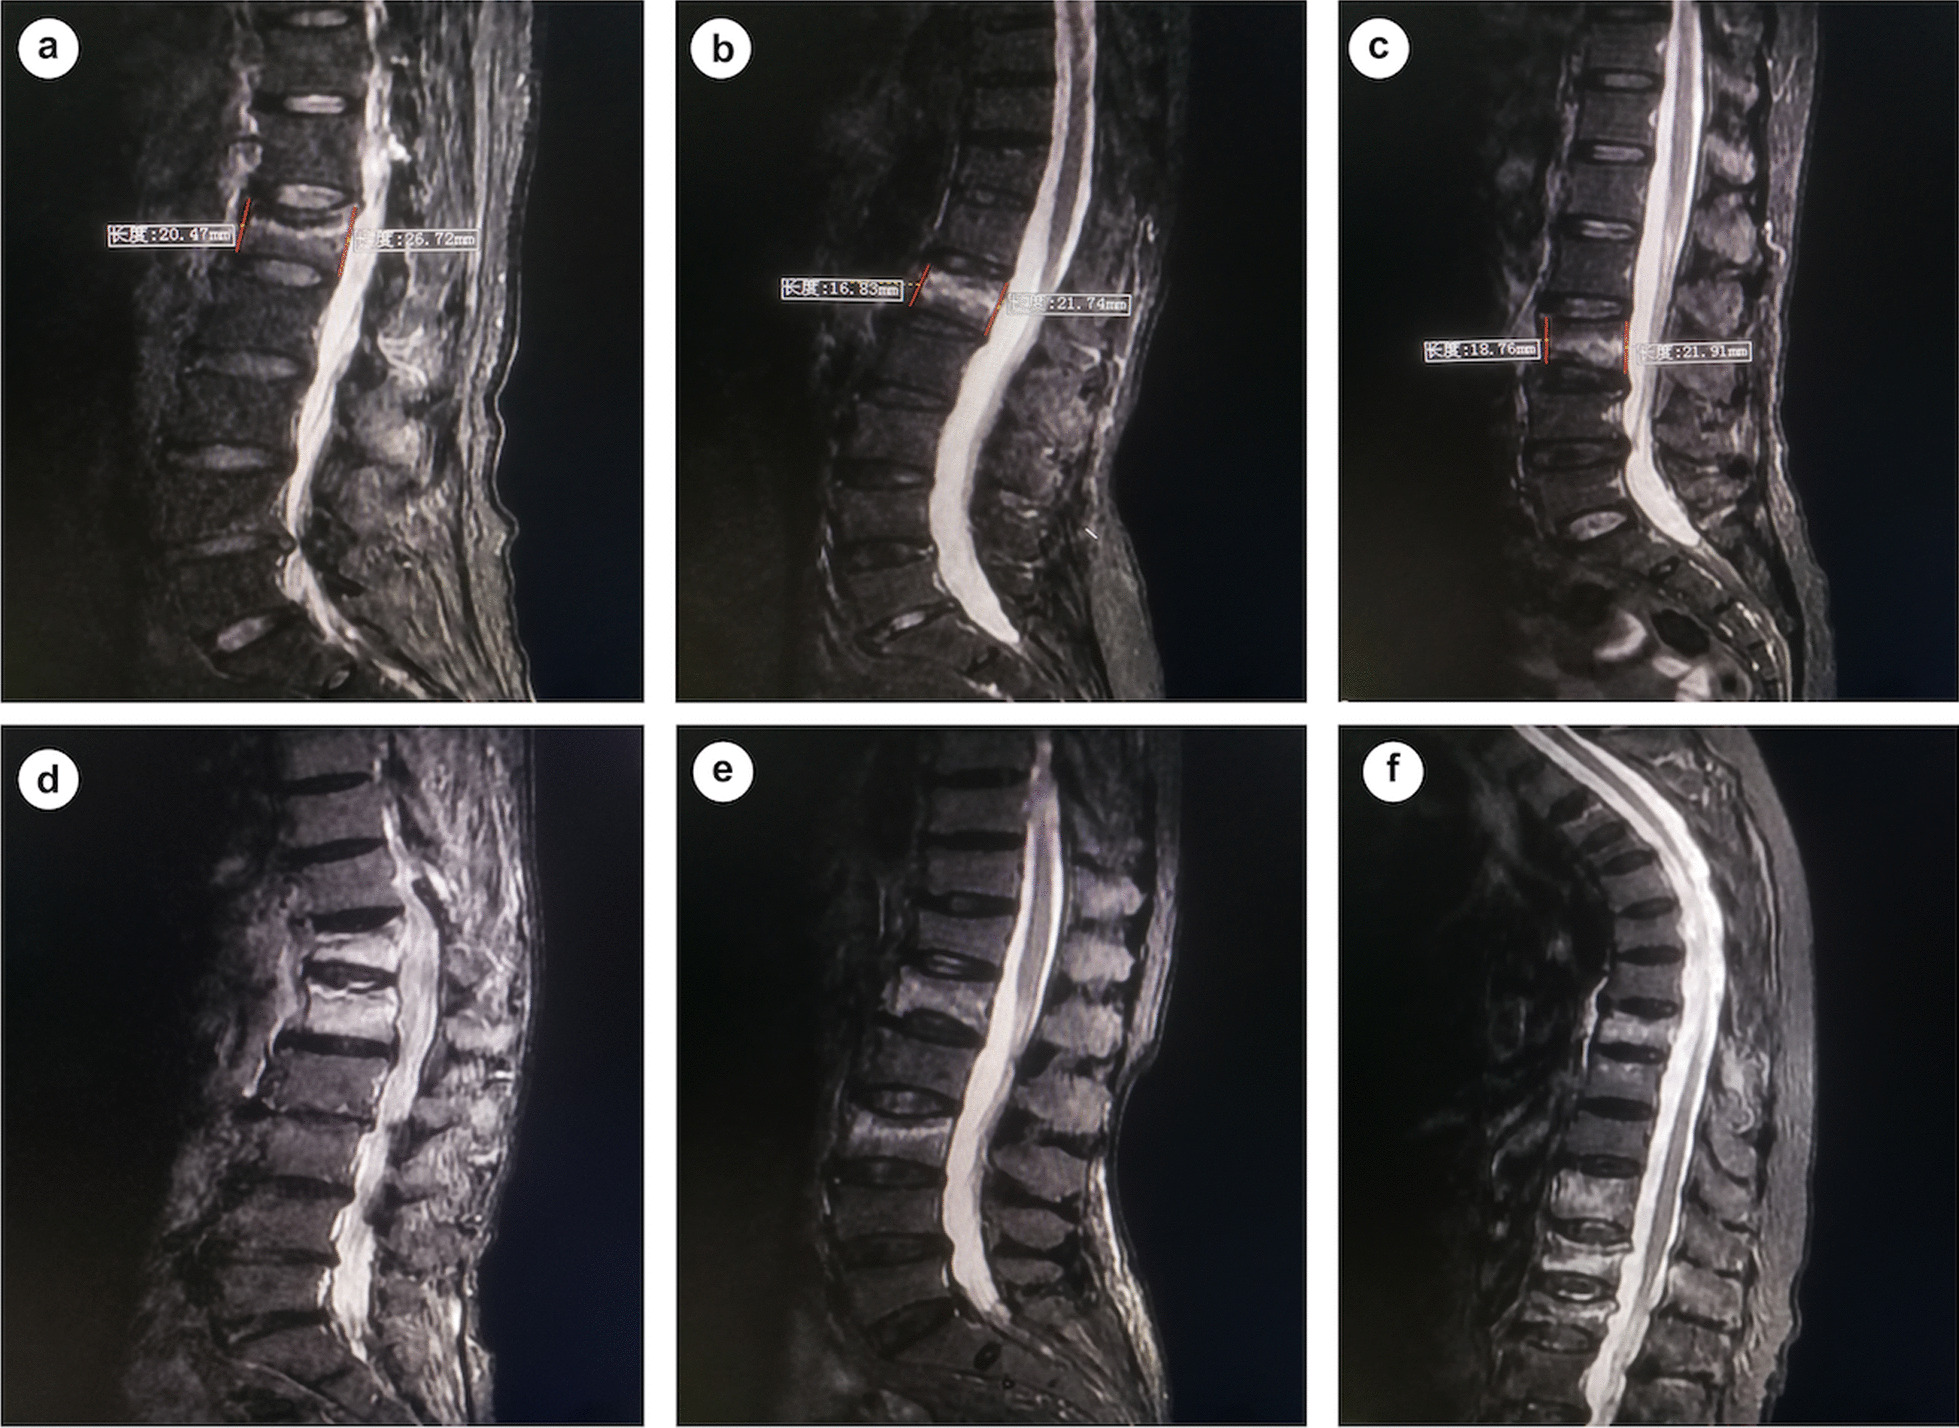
Radiographic characteristics of single and multiple osteoporotic vertebral compression fractures. The sagittal T2-weighted fat suppression MR imaging of single (a–c) and multiple (d–f) vertebral compression fractures. a A 78-year-old male complaining back pain for 5 days after fell on ground showed vertebral bone marrow edema in the cranial half of L1 vertebrae. The anterior and posterior height (red line) of fractured vertebrae was measured to quantify the degree of vertebral compression. b A 79-year-old female without spine trauma complained back pain for 1 week and showed diffused type of vertebral bone marrow edema in L1. c A 66-year-old female complained back pain for 4 days after heavy lift injury showed vertebral bone marrow edema in the caudal half of L3. d Two acute vertebral compression fractures in adjacent (T12 and L1) were detected in a 81-year-old male complaining back pain for 1 month without spine trauma. e Two acute vertebral compression fractures (L1 and L3) intermittent with one intact vertebrae (L2) were detected in a 74-year-old female complaining back pain for 1 month without spine trauma. f Four acute vertebral compression fractures (T8, T11, T12, and L1) with three in adjacent were detected in a 81-year-old female complaining back pain for 2 weeks without spine trauma
